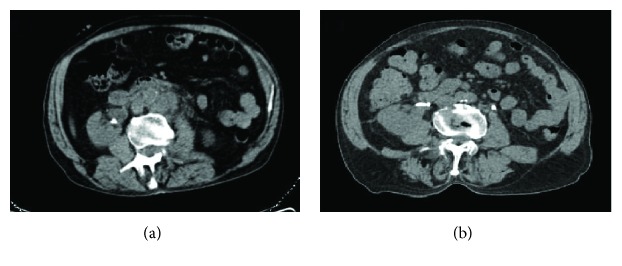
Computed tomography (CT) images (a) before and (b) after 3 administrations of atezolizumab showing a significant decrease in the size of lymph nodal metastasis.
